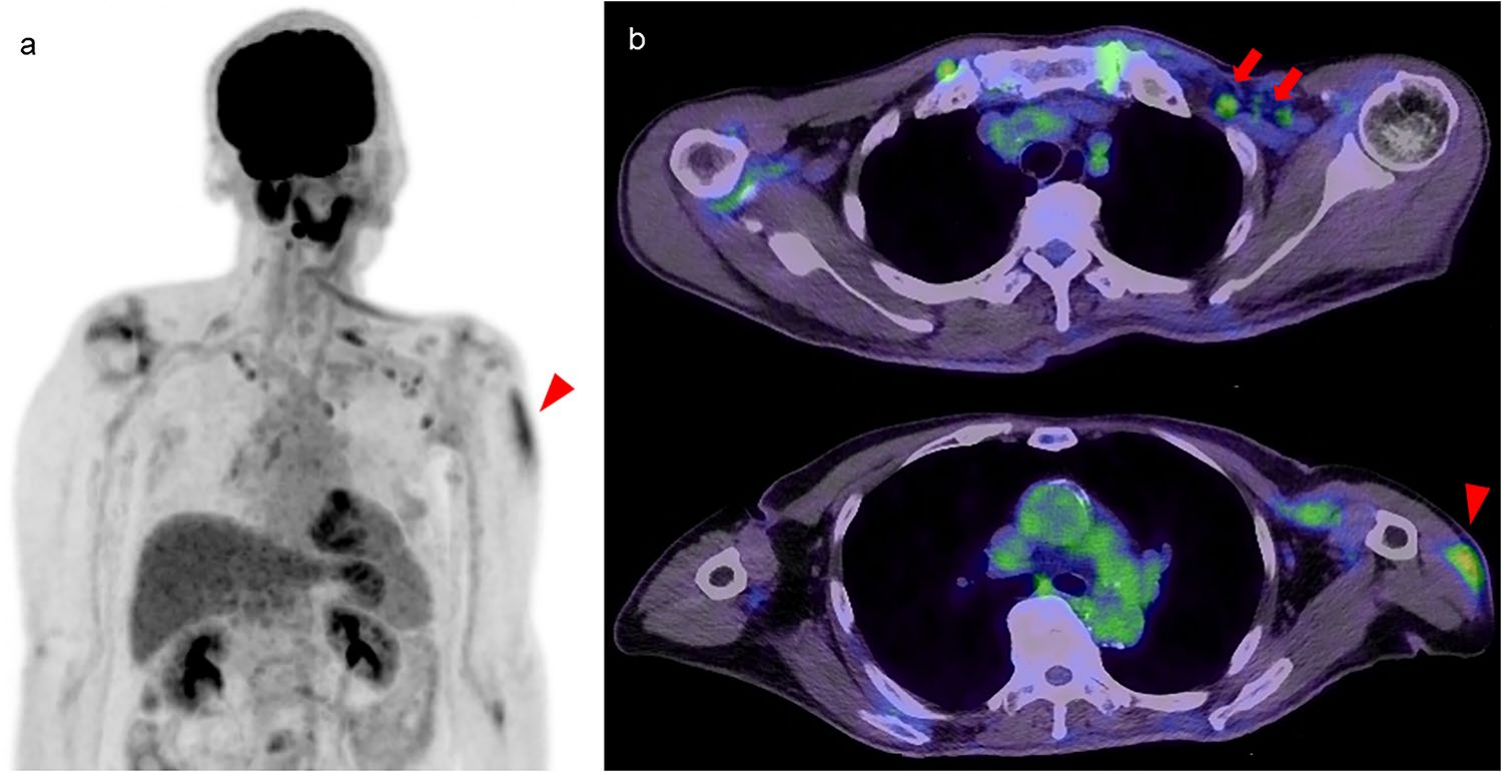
a FDG-PET MIP image. b Axial FDG-PET/CT images. Moderate FDG uptake is confirmed in the deltoid muscle (arrowhead), indicating inflammatory change following administration of the COVID-19 vaccine. FDG uptake in axillary lymph nodes can also be confirmed with this procedure (arrow)
